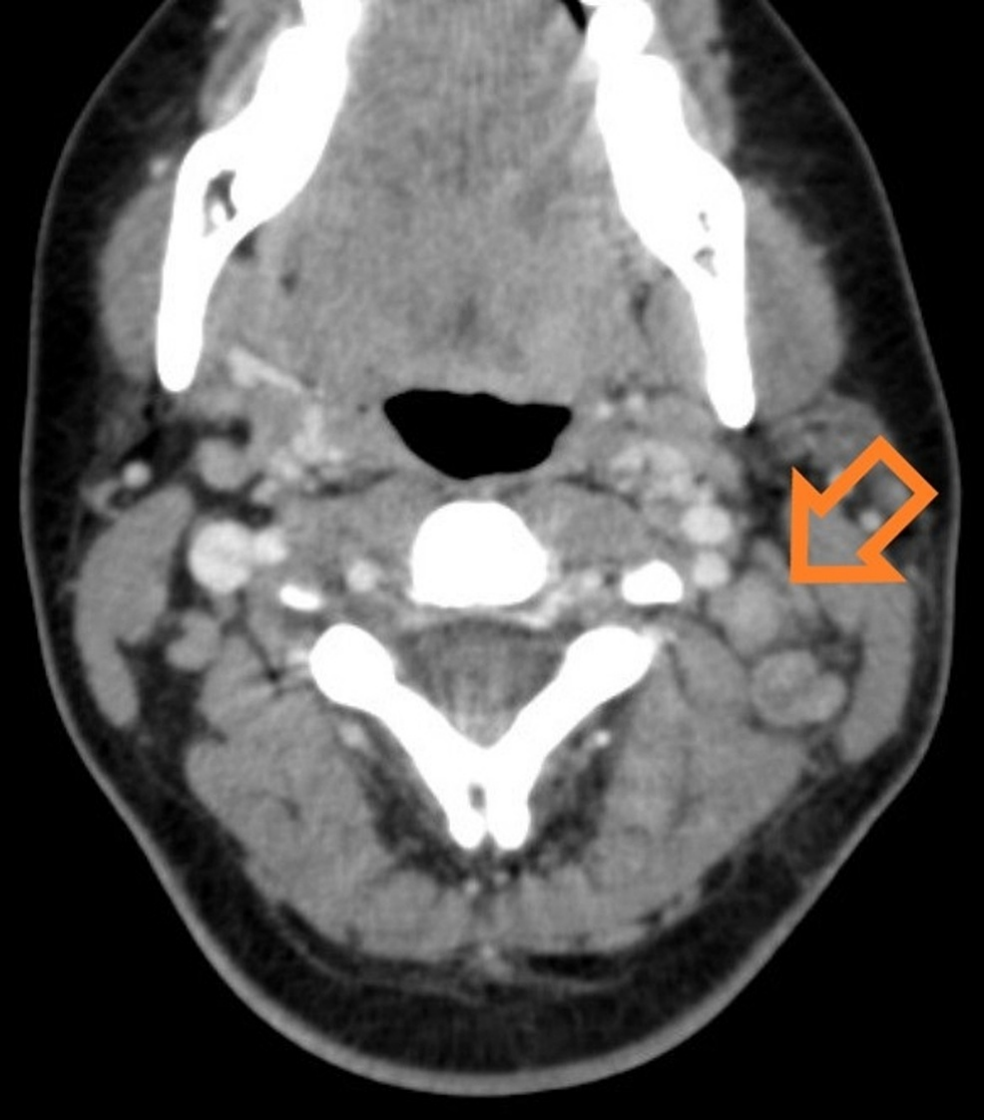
Computed tomography showing multiple cervical lymphadenopathies Orange arrow showing lymphadenopathies
